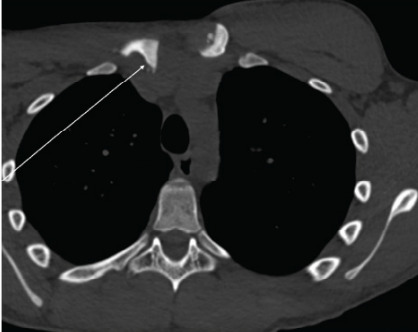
Axial computed tomography without contrast showing posterior displacement of the right medial clavicle relative to the left clavicle, noted by arrow.
